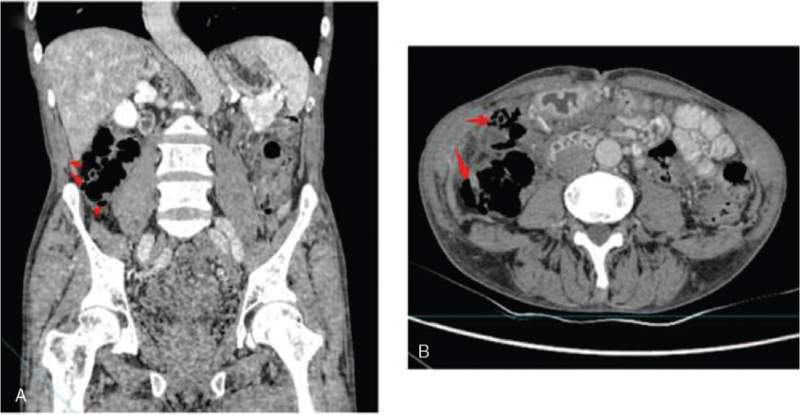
Coronal plus axial abdomen CT showing the intramural gas in the ascending colon.
